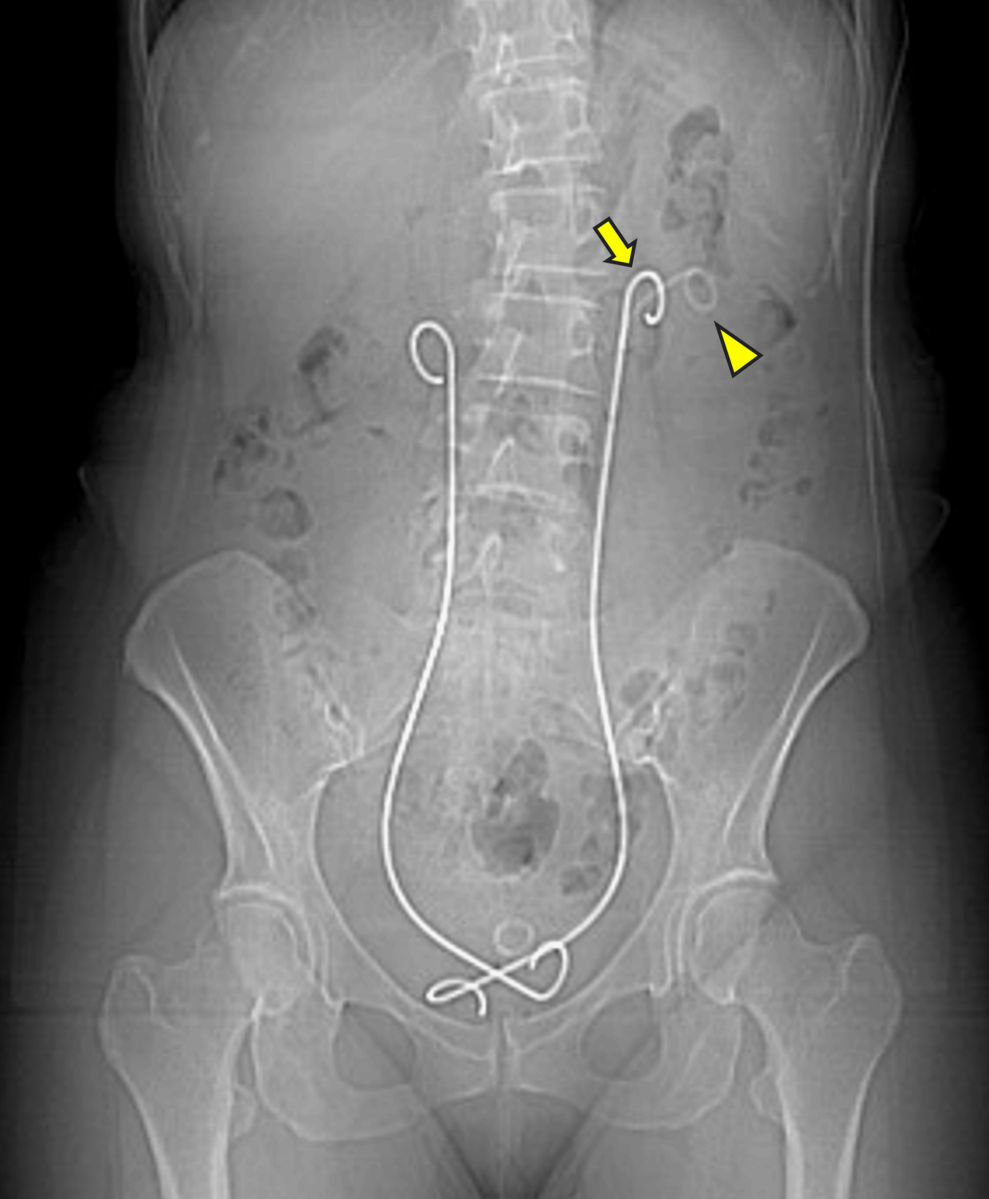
A plain abdominal radiograph at presentation to our institution. Metallic ureteral stent (arrow); polyurethane double-J (DJ) ureteral stents (arrowhead).
